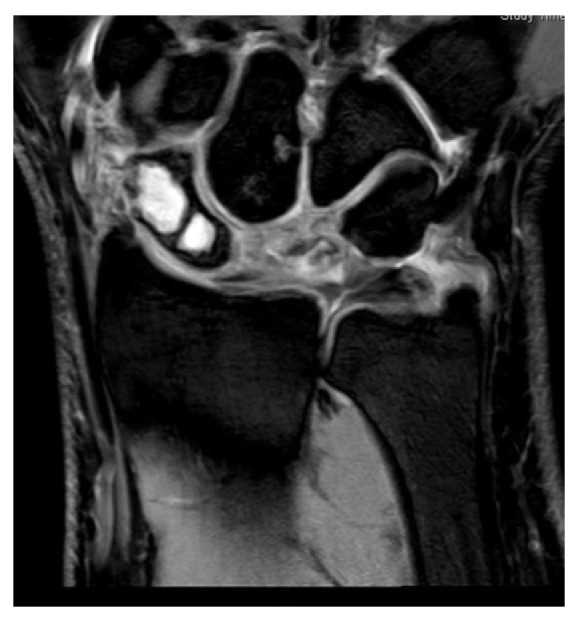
MRI: Giant interosseous ganglion of the scaphoid with cortical bone disrupture, lunate collapse, no severe chondropathy fossa lunata, and capitate.
